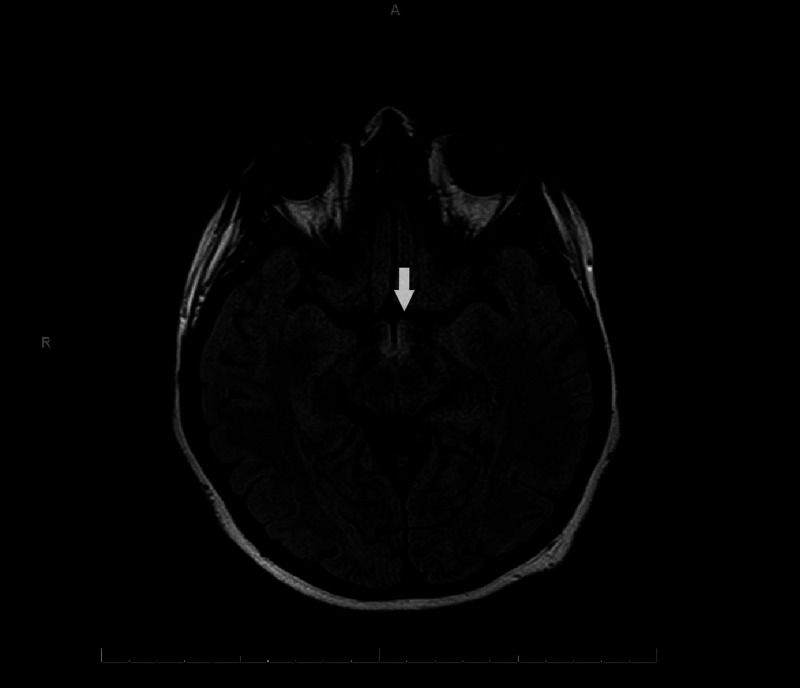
Axial T2 fluid-attenuated inversion recovery (FLAIR) image showing hyperintensity at mammillary bodies.
